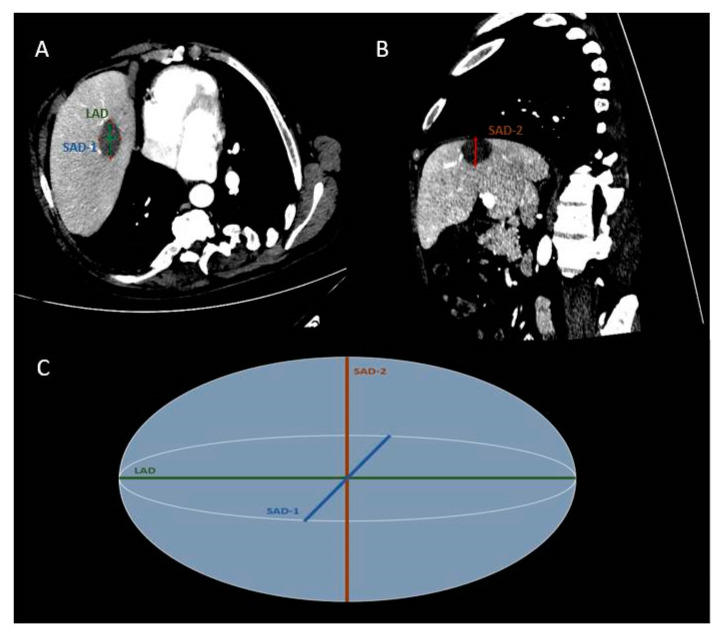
CT scan of a 54-year-old man showing a colorectal cancer metastasis in liver segment VIII post microwave ablation (MWA). Enhanced transverse (A) and parasagittal (B) CT images. Simplified scheme of MWA zone (C). The short-axis diameters (SAD-1,-2) are orientated perpendicular to the long-axis diameter (LAD).
